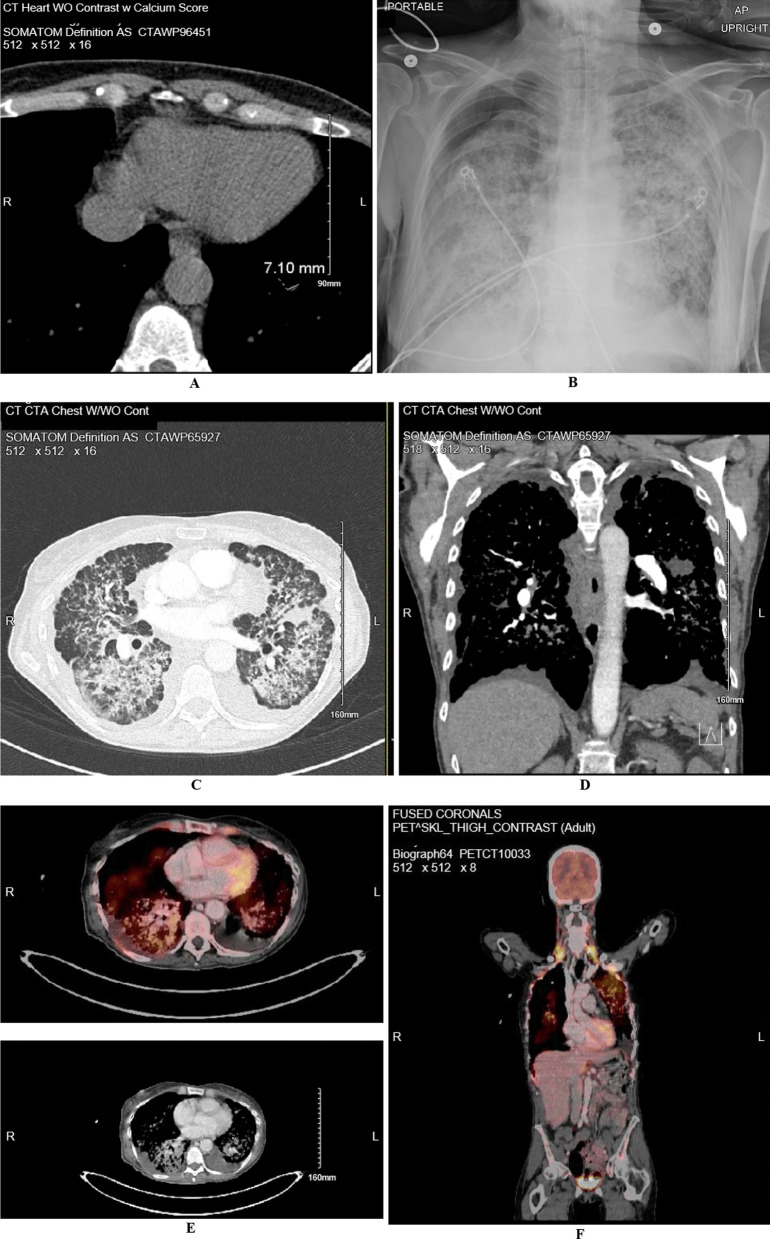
A, B Computed tomography (CT) without contrast with calcium score performed 3 year prior to admission for evaluation of coronary artery disease showing a score of zero in all branches and a 7 mm nodule in the left lower lobe. B Day of admission 1-view chest X-ray (CXR) showing a right pneumothorax with a 3.2 cm pleural separation, prominent diffuse bilateral interstitial alveolar opacities. C, D CT chest with and without contrast showing diffuse nodular opacities throughout the lungs with areas of coalescence, in addition to small bilateral pleural effusions. E Positron emission tomography (PET) showing diffuse hypermetabolic interstitial and airspace abnormality of the lungs without lymphadenopathy (or distant lesions), in addition to right hydropneumothorax and left pleural effusion
